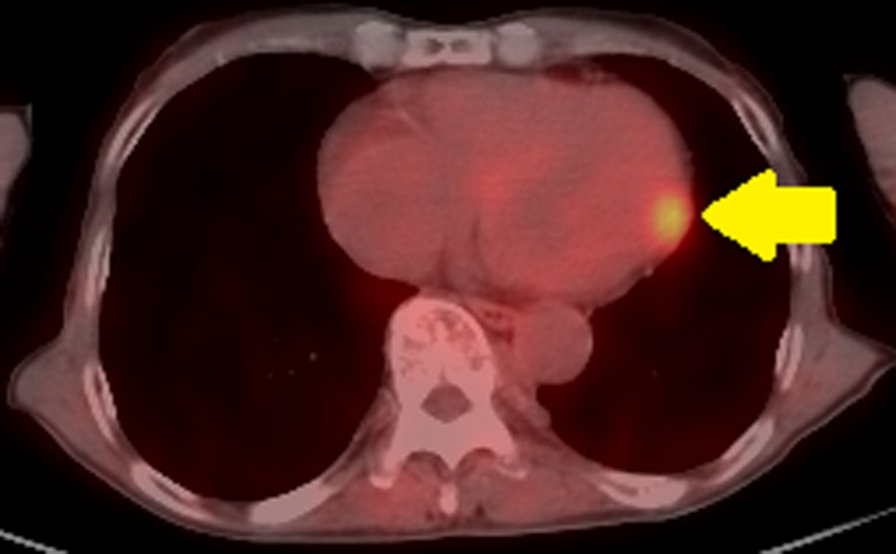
PET showed a high degree of FDG accumulation in the left ventricular myocardium (arrow)
